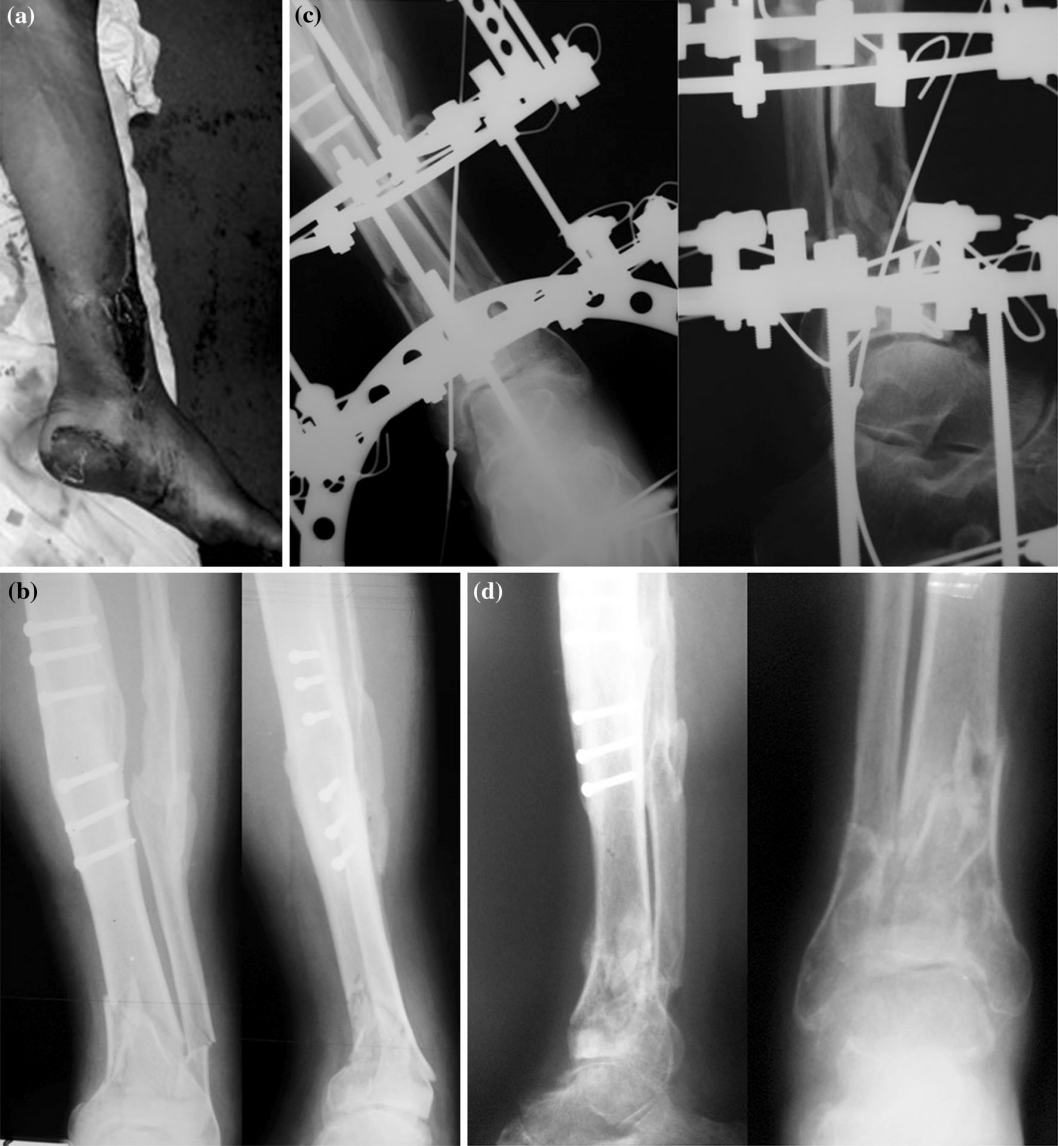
An example of a 56-year-old male with a 3B open Pilon type III fracture- treated with Ilizarov fixator. a Clinical picture, b preoperative X-rays, c X-rays 6 weeks post-operatively, d nine months post-operatively the fracture healed, and the frame was removed
